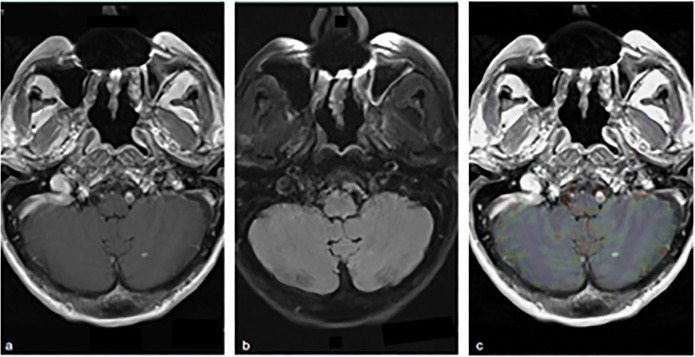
Disease recurrence in L cerebellar hemisphere. (A) T1 axial 1 GD, (B) T2 FLAIR, and (C) T1 OLEA. FLAIR, fluid-attenuated inversion recovery.
